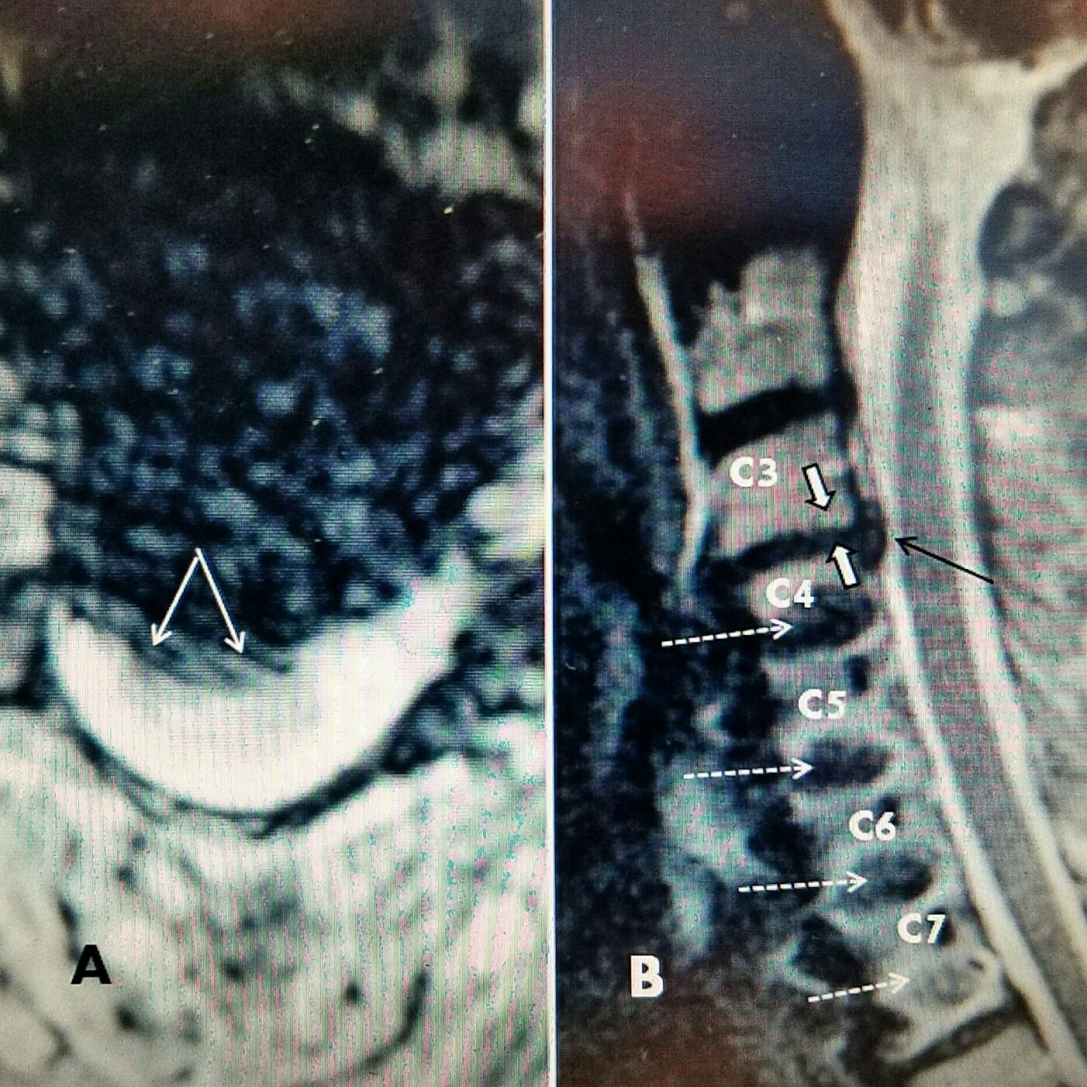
Patient #3 C3-4 herniated disc above previous ACDF and plating C4-C7 A: Axial MRI: C 3-4  midline and right lateral herniated disc (two solid white arrows). B: Sagittal T2 MRI shows 3 mm herniated C 3-4 disc (black arrow) with some moderate posterior disc space narrowing (white arrows). Screws at C4, 5, 6, 7 (dotted arrows). ACDF: Anterior cervical discectomy and fusion; MRI: Magnetic resonance imaging.
